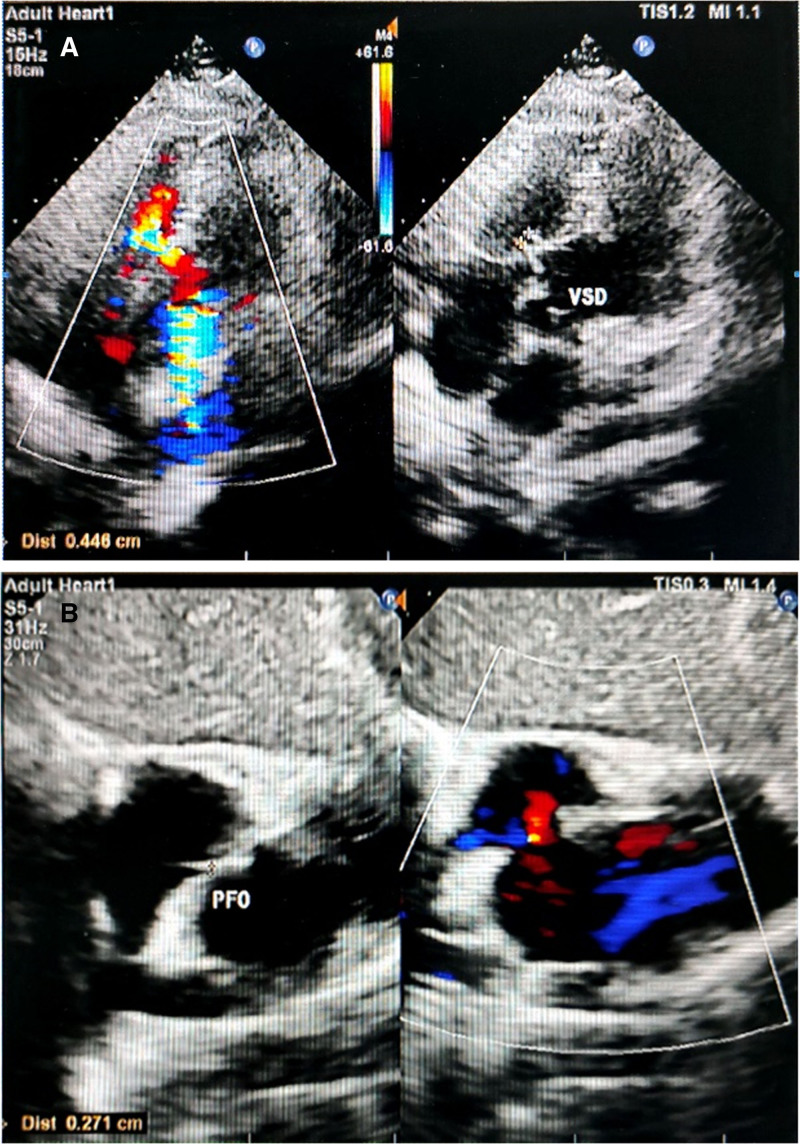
Pre-procedure transthoracic echocardiography of the patient. (A) Ventricular septal defect; (B) patent foramen ovale.
